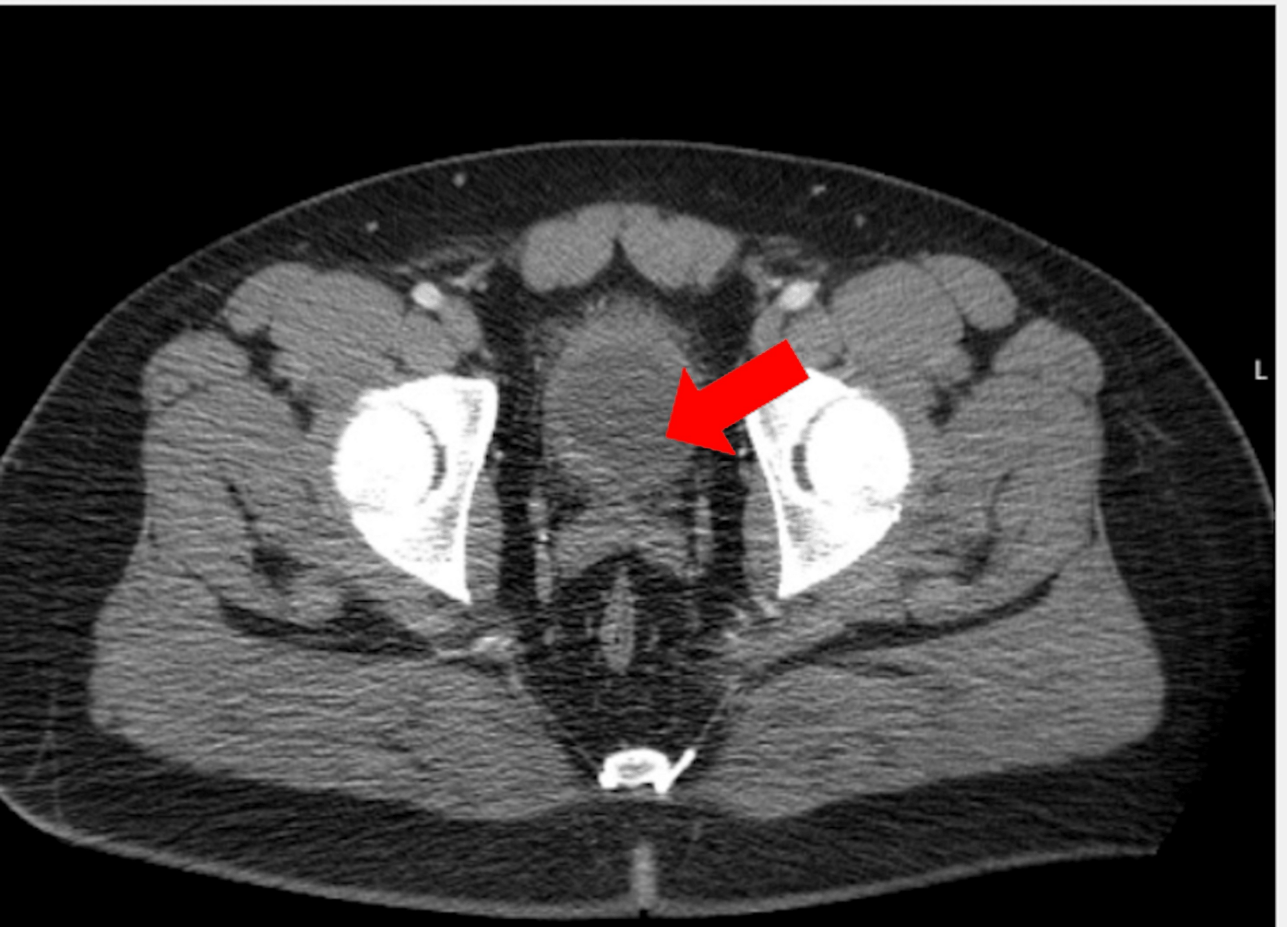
Contrast axial abdominopelvic CT scan demonstrating a bladder mass suggestive of malignancy. The red arrow in the figure is pointing at diffuse wall thickening involving the anterior aspect of the bladder with subtle pre-vesical fat stranding suggestive of malignancy.
